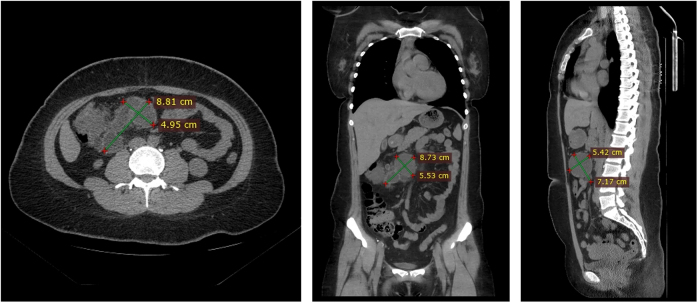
Post-operative CT scans of mesenteric cysts. Post-operative CT scan demonstrates a substantial reduction in cyst size, measuring 8.73 cm × 5.53 cm, following thoracic duct decompression and nutritional management, indicating successful treatment of the chylous mesenteric cyst.
